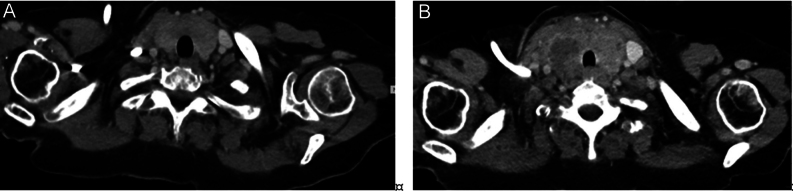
(A) CT-scan performed at the end of July with contrast agent shows the thyroid gland slightly enlarged with slight inhomogeneity of the structure. (B) CT-scan performed with contrast agent, 5 days later, shows the enlarged thyroid gland with a nonhomogeneous structure, with hypodense areas, which surrounds the trachea.
